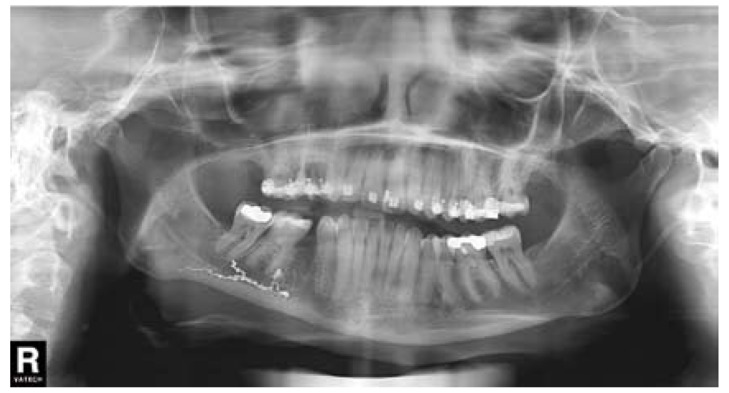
Panoramic radiograph revealing the presence of radiopaque material (endodontic sealer) within the mandibular canal. Tooth 45 has been extracted.
